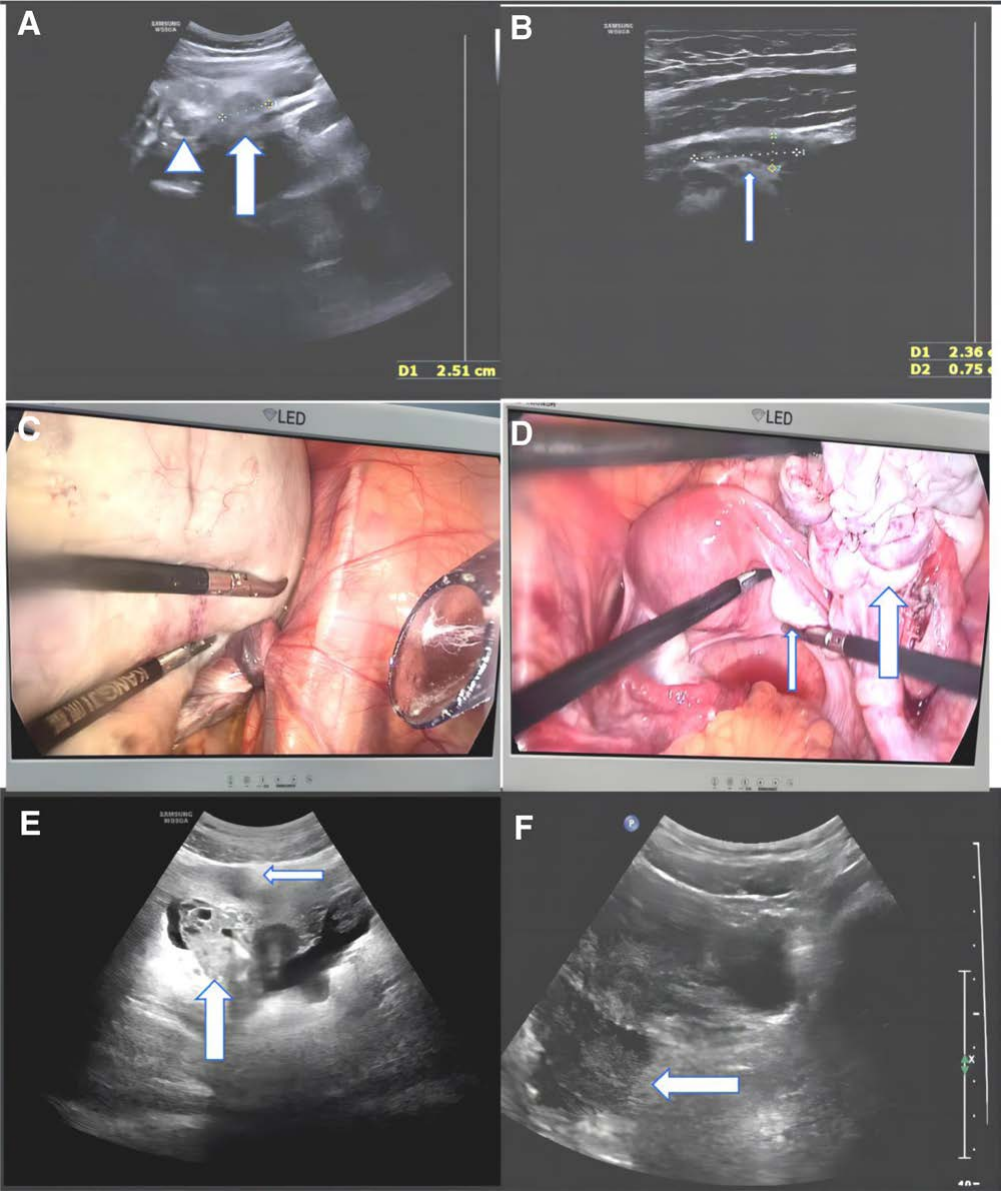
Preoperative ultrasound image showed a hyper-echoic ovary appearance of 2.5 × 1.4 cm size (A) and a normal ovary appearance of 2.4 × 0.8 cm size (B) on the right adnexal area. Intraoperative findings showed the accessory ovarian tumor with torsion (C) and the eutopic right ovary (D). Postoperative ultrasound image showed hypo-echoic eutopic ovary and hyper-echoic accessory ovary 20 d after the surgery, antral follicles were found both in them (E). Ultrasound result 4 mo after the surgery: Two hypo-echoic findings on the right side adnexal area: echoic eutopic ovary and accessory ovary (F). The thin arrow, the eutopic right ovary. The thick arrow, the accessory ovary. The arrowhead, the whirlpool sign.
